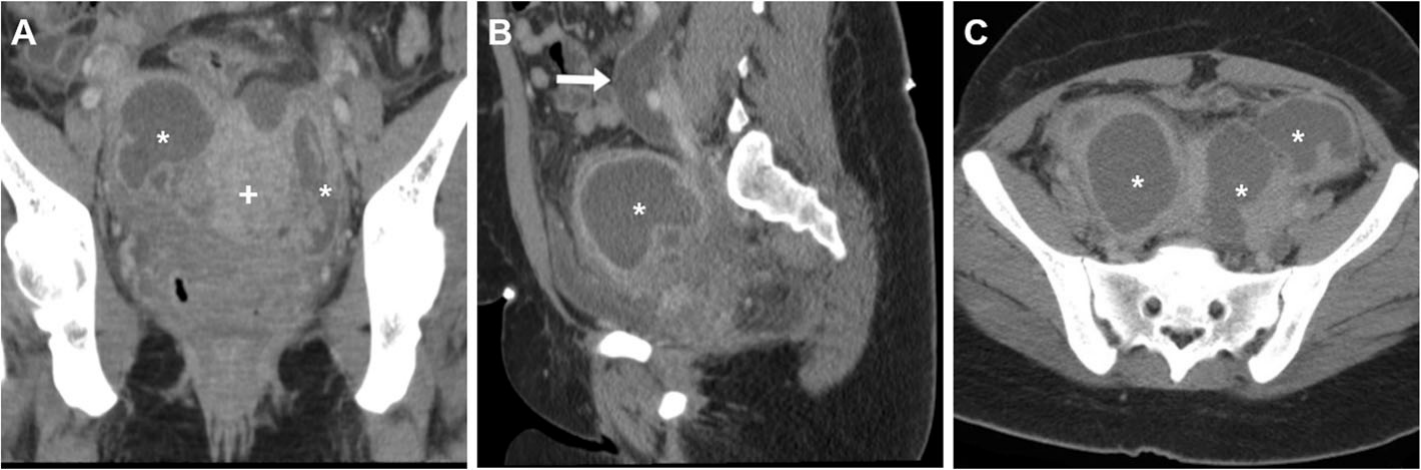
a–c Multiplanar CT images of an extensive PID in a 27-year-old African woman, complicated by multiple, confluent abscesses (asterisk) occupying the entire pelvis, that compress the uterus (plus sing) on the midline. Note hydronephrosis (arrow in b). Clinical and radiological suspicion of actinomycosis was not supported by microbiology samples
